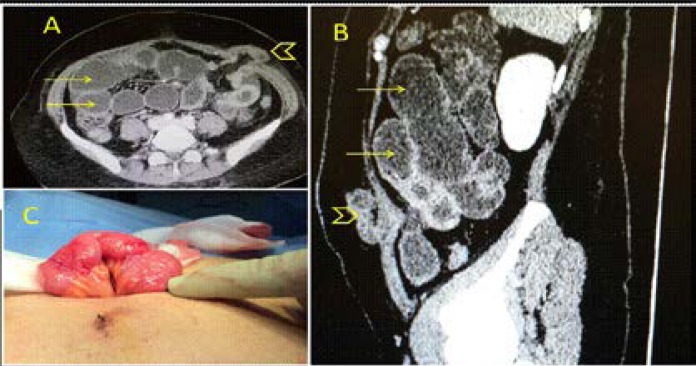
A 30-year-old female had a laparoscopic dermoid cystectomy. She developed abdominal pain, distension and vomiting 3 days after surgery. Abdominal CT scan with intravenous and oral contrast showed (A–B) distended small bowel loops (yellow arrows) and an incarcerated bowel loop in one of the ports (arrow head). The bowel was viable (C), the port incision was extended, the bowel was reduced and the hernia was repaired (Courtesy of Dr Islam Sidky, Consultant Obstetrician and Gynecologist, Department of Obstetrics and Gynecology, Tawam Hospital, Al-Ain, United Arab Emirates).
